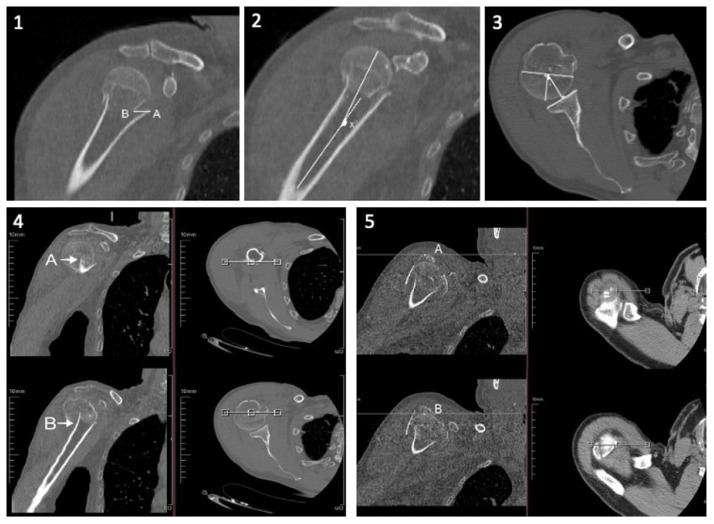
Measurement of parameters on computed tomography (1-medial hinge, 2-cephalodiaphyseal angle, 3-cephaloglenoid angle, 4-medial hinge impaction, 5- tubercle displacement).
